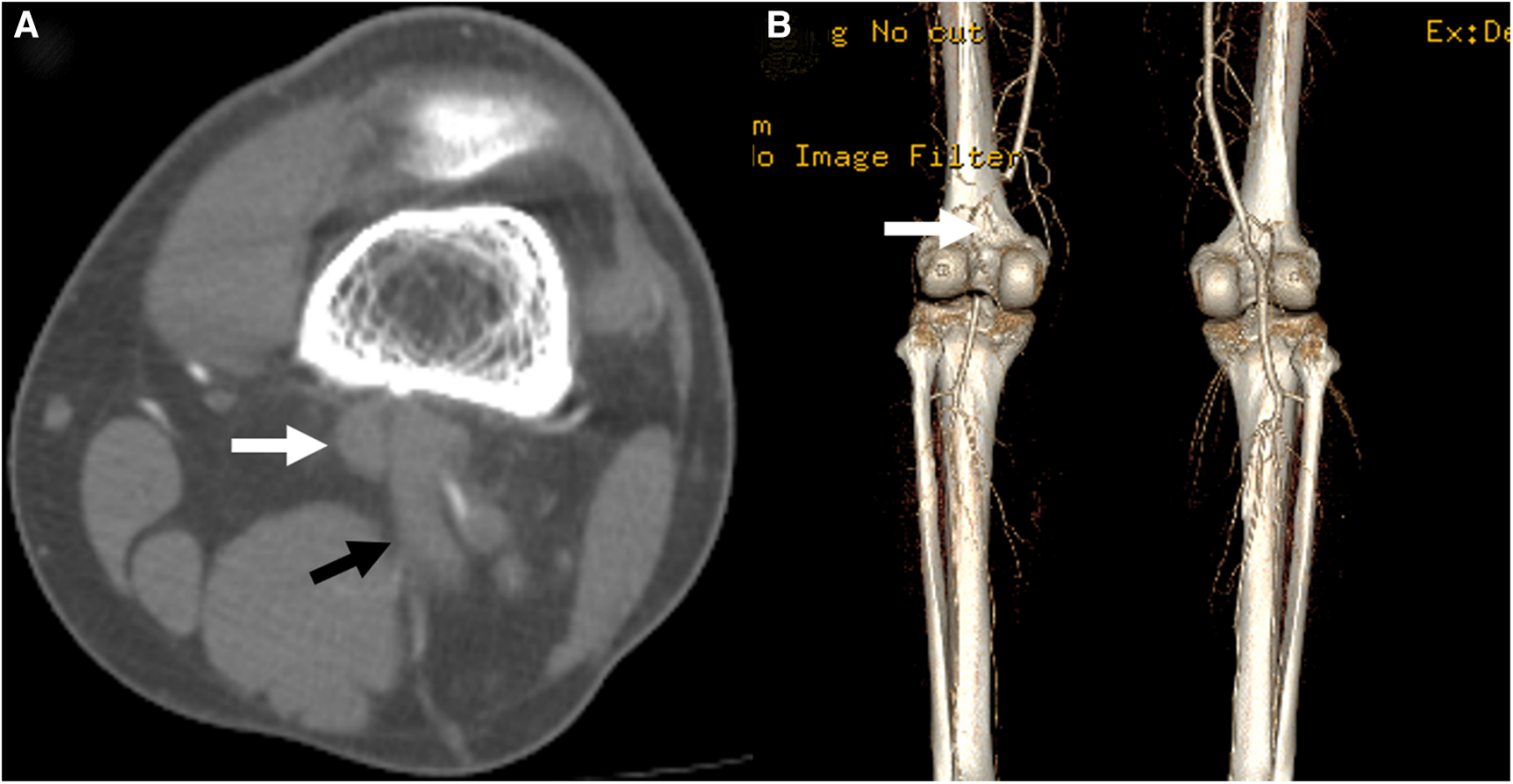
A computed tomography scan conducted at the time of the second presentation showed occlusion of the popliteal artery (*white arrow*) in the axial (A) and 3-dimensional reconstruction (B, posterior view) images. The anomalous insertion of the medial head of the gastrocnemius muscle between the popliteal artery and vein was also seen (*black arrow*).
